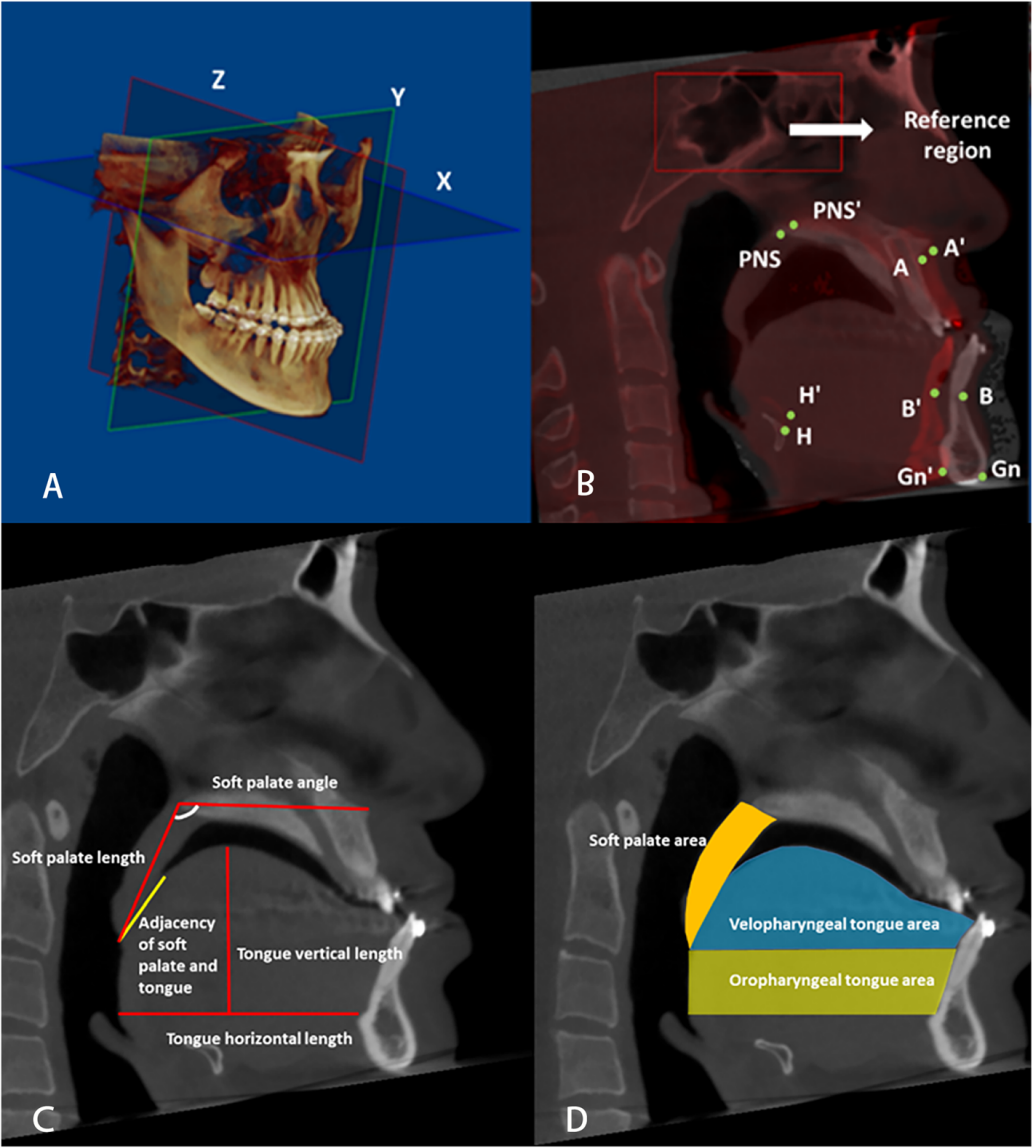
Three-dimensional coordinate system and image superimposition of the CBCT. A. Three-dimensional coordinate system. B. Superimposition of preoperative and postoperative CBCT. The red box stood for the reference region. The definition landmarks were given in Table 2. C. Linear measurements of soft palate and tongue. D Area measurements of soft palate and tongue
